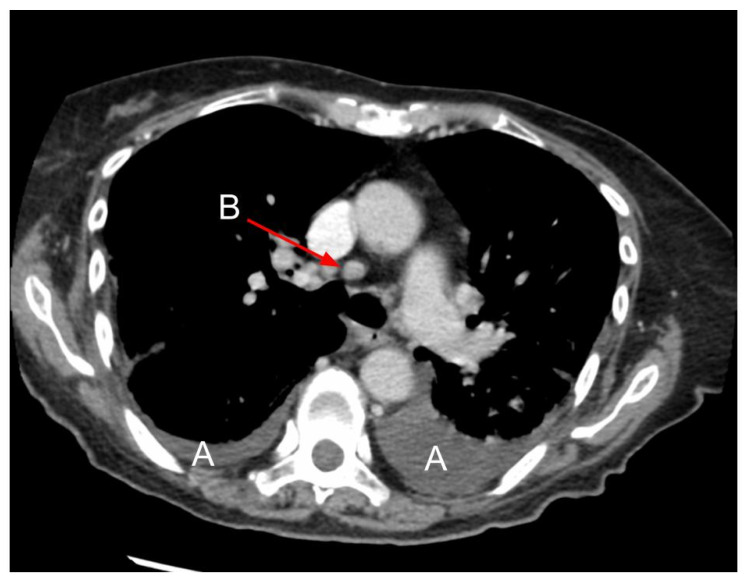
Mediastinal lymphadenopathy in CT. A: Bilateral pleural effusion; B: Lymphadenopathy of pretracheal lymph nodes (4R). Figure obtained with permission from the 2nd Department of Radiology, Medical University of Warsaw.
